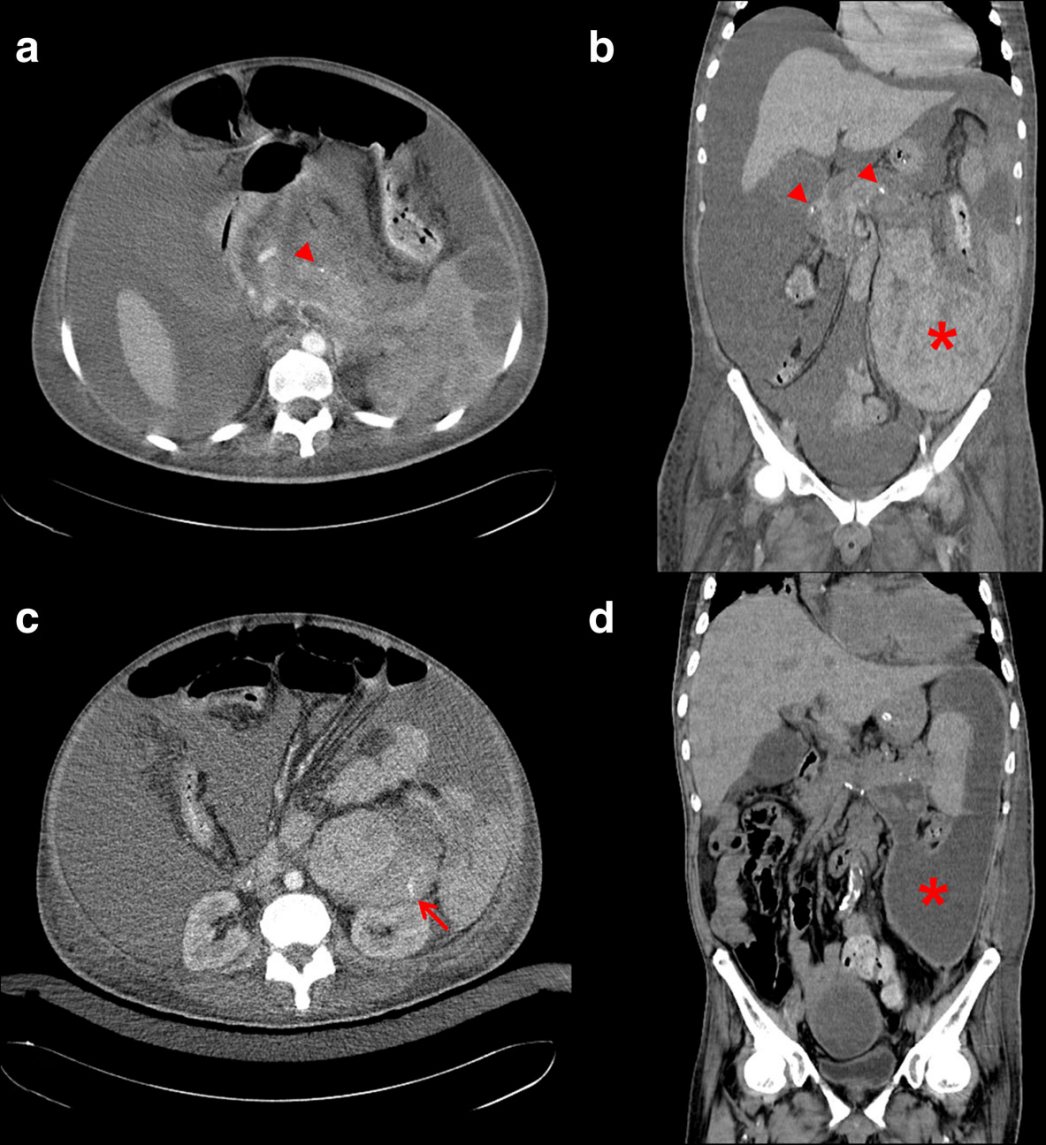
a An axial view of the contrast-enhanced abdominal CT shows in the pancreas a heterogeneous pattern and some calcified spots (*arrowhead*) that are compatible with pancreatitis. b A coronal view shows intrapancreatic calcifications (*arrowheads*) and one high dense lesion, with a huge hematoma, which occupied the perisplenic and pararenal retroperitoneal space and extended to the lower left abdomen (*asterisk*). c A high-resolution image shows one active contrast medium extravasation in the pararenal region (*arrow*). There was also abundant ascites, caused by advanced hepatic failure and right heart failure. d A coronal view of contrast-enhanced abdominal CT 2 months later showed fluid accumulated in the space previously occupied by the hematoma (*asterisk*), which is compatible with chronic pancreatitis with one huge and extended pseudocyst, because the fluid had an extremely high concentration of pancreatic enzymes
